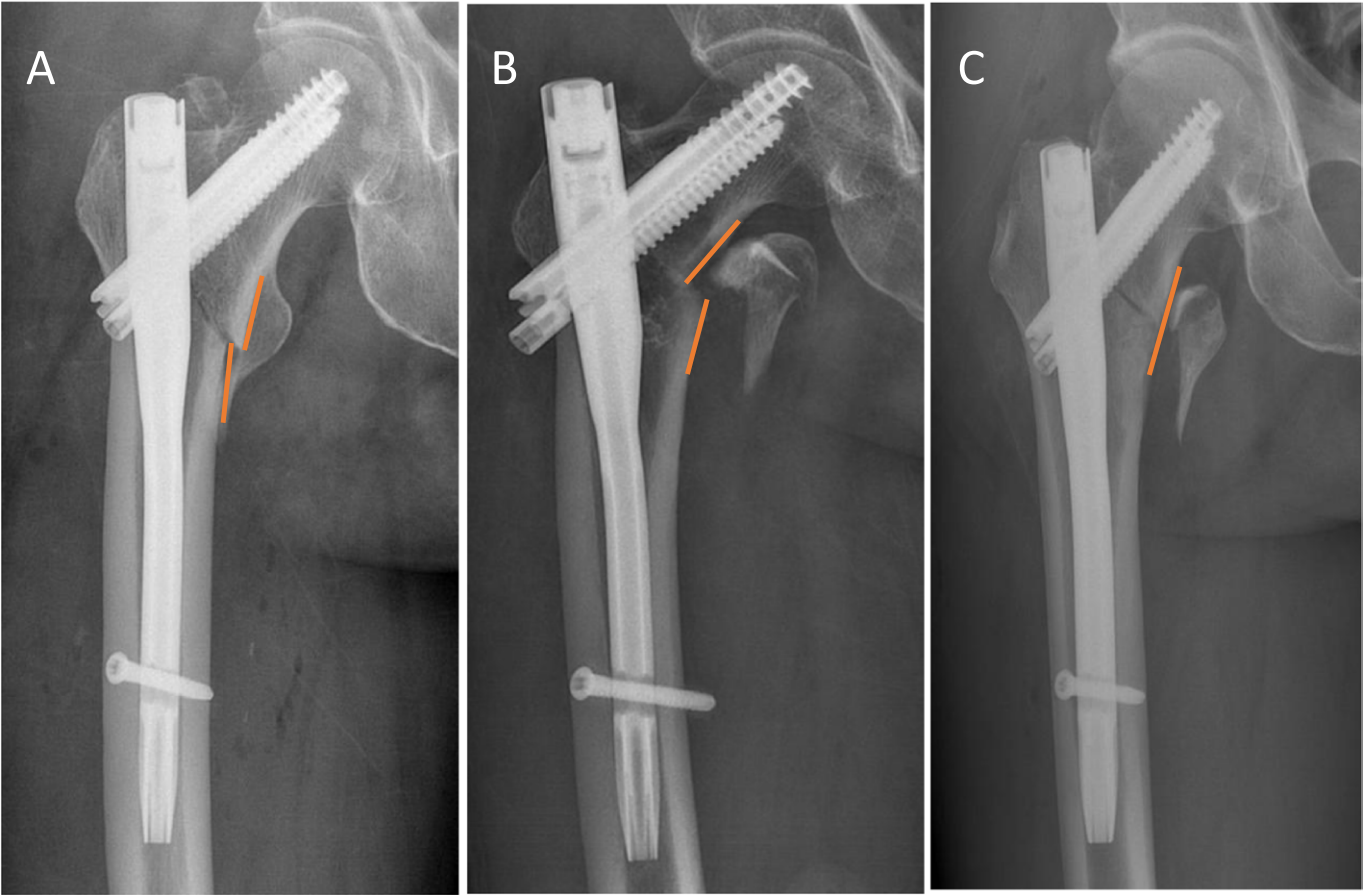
Postoperative X-ray of intertrochanteric fractures. A X-ray shows positive medial cortical support, meaning that the inferior edge of the medial cortex of the femoral head-neck fragment is medial to the superior edge of the medial cortex of the femoral shaft with a displacement of less than one cortical thickness. B X-ray shows negative medial cortical support, meaning that the inferior edge of the medial cortex of the femoral head-neck fragment is lateral to the superior edge of the medial cortex of the femoral shaft, regardless of displacement distance. C X-ray shows neutral medial cortical support, meaning that the medial cortex of the head–neck fragment and the femoral shaft are in smooth contact
